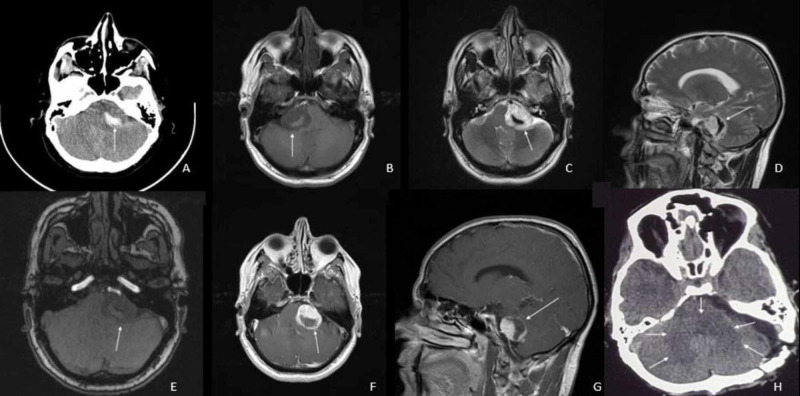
Patient's Preoperative Imaging (A) Brain CT shows an extra-axial lesion at the left CPA region with intralesion hematoma. (B and C) Axial T1- and T2 -weighted images, respectively, show extra-axial lesion, which was hypointense on T1-weighted images and hyperintense on T2-weighted images. Intralesional hematoma is iso- and hypointense on T1- and T2-weighted images, respectively. (D) Sagittal T2-weighted image. (E) Axial MRI source image. (F and G) Contrast T1-weighted axial and sagittal images show homogenous avid tumor enhancement. (H) Contrast CT show postoperation changes and tumor resection with retrosigmoid approach.
